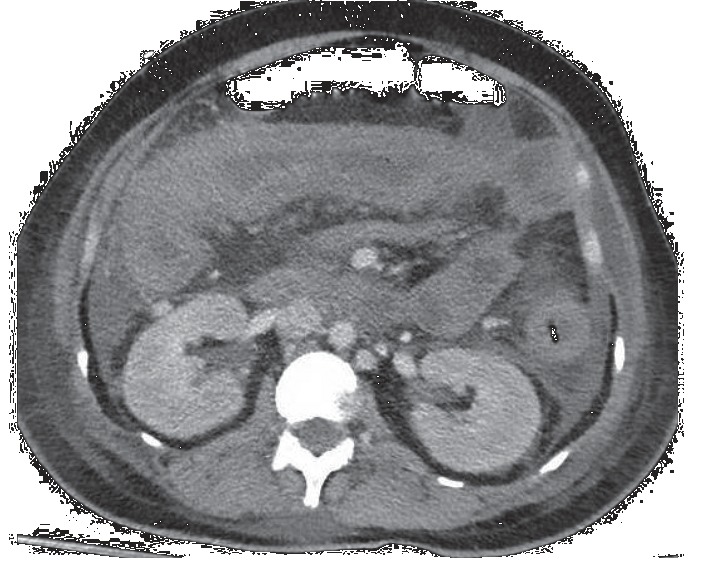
Contrast-enhanced abdomen computed tomography with oedematous thickening of the caecal and colonic mucosa.
